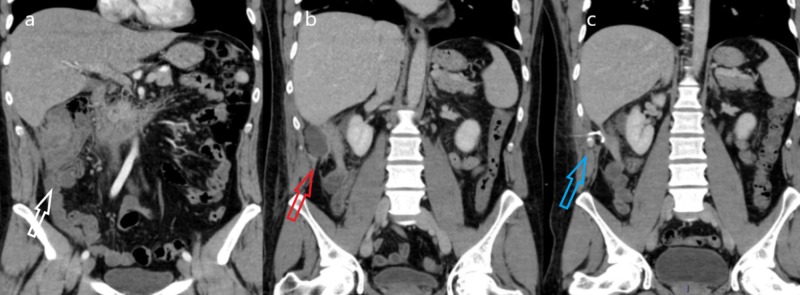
Coronal IV-contrast abdominal CT scans. a) Image shows the cecum with circumferential stranded fat signaled by the white arrow, notice the ascended position of the cecum. b) The red arrow signals a hypodense liquid-attenuated collection adjacent to the cecum. c) This image corresponds to the CT scan after drain placement signaled by the blue arrow, notice the volume reduction of the collection. IV: intravenous
